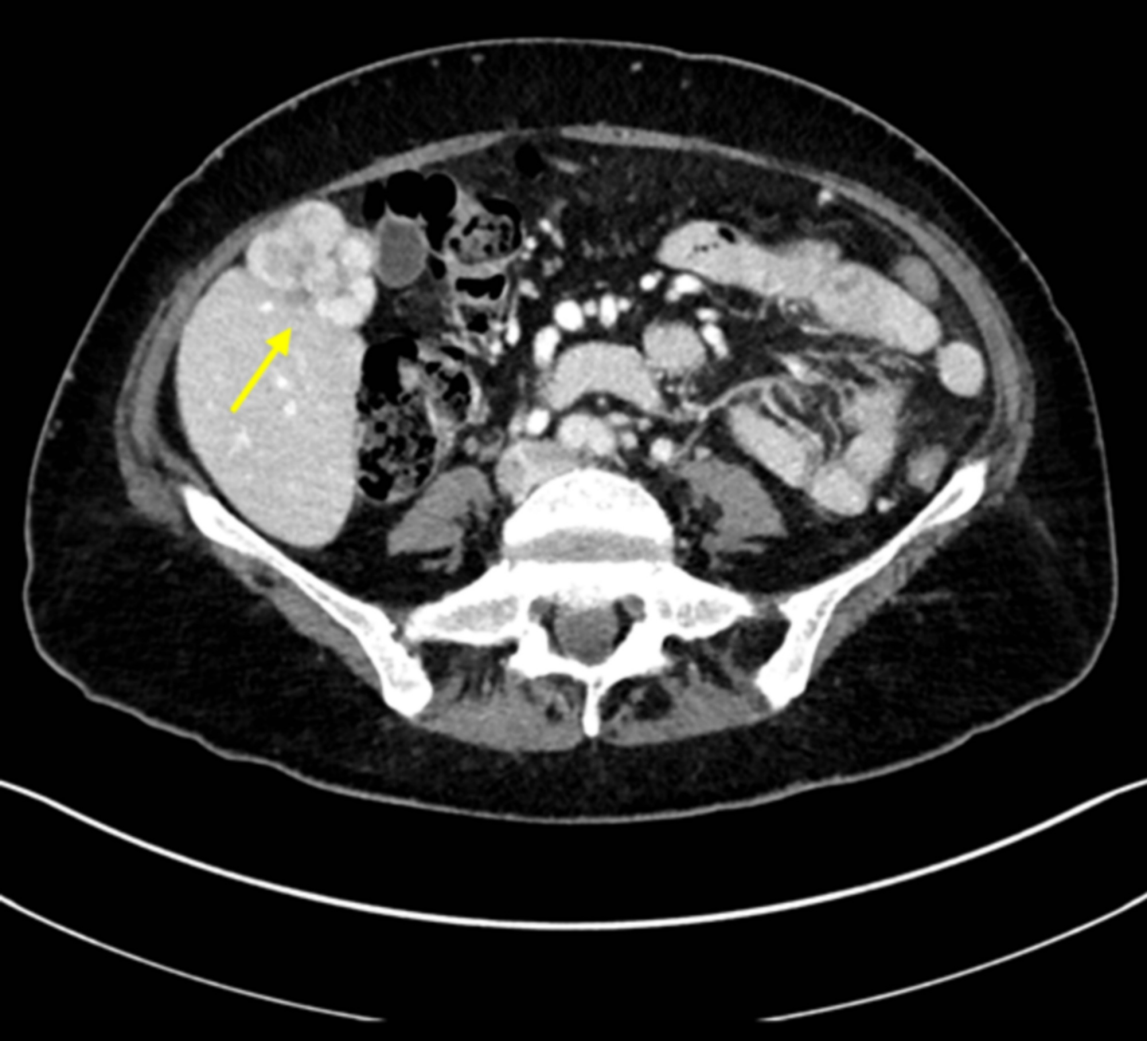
Contrast-enhanced abdominal CT CT: computed tomography CT image of a 38-year-old female on oral contraceptives. Portal venous phase contrast-enhanced axial CT image demonstrates a multilobulated mass with heterogeneous enhancement. This was a biopsy-proven adenoma
